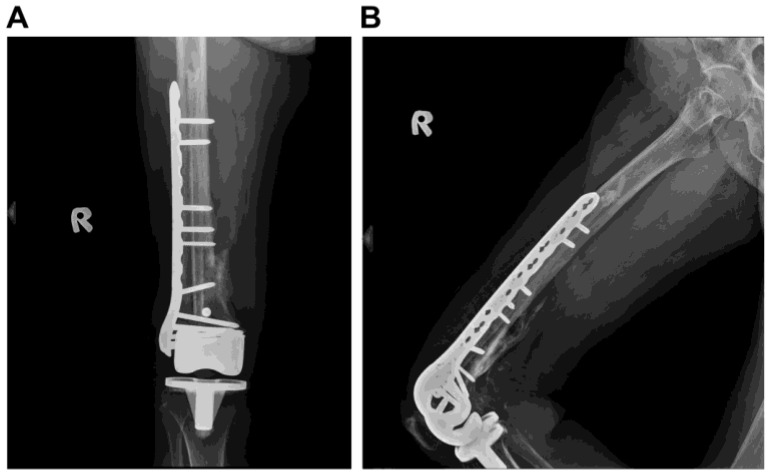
Radiographic images taken 4 months after the surgery. (A) Anteroposterior (AP) view of knee. (B) Lateral view of knee. The patient achieved a solid bone union.
